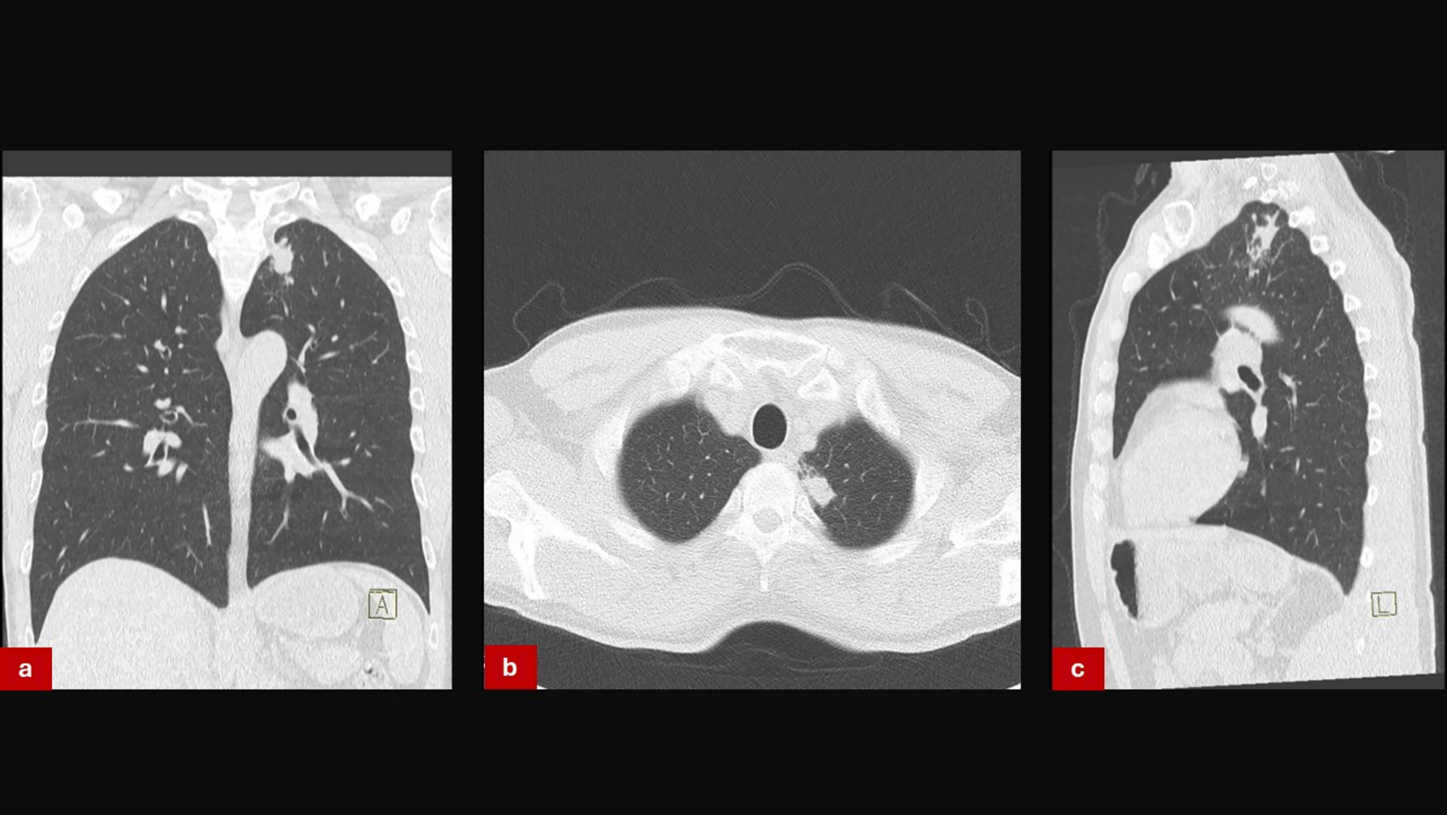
(a–c) CT‐scan of solitary mass in left upper lobe.
